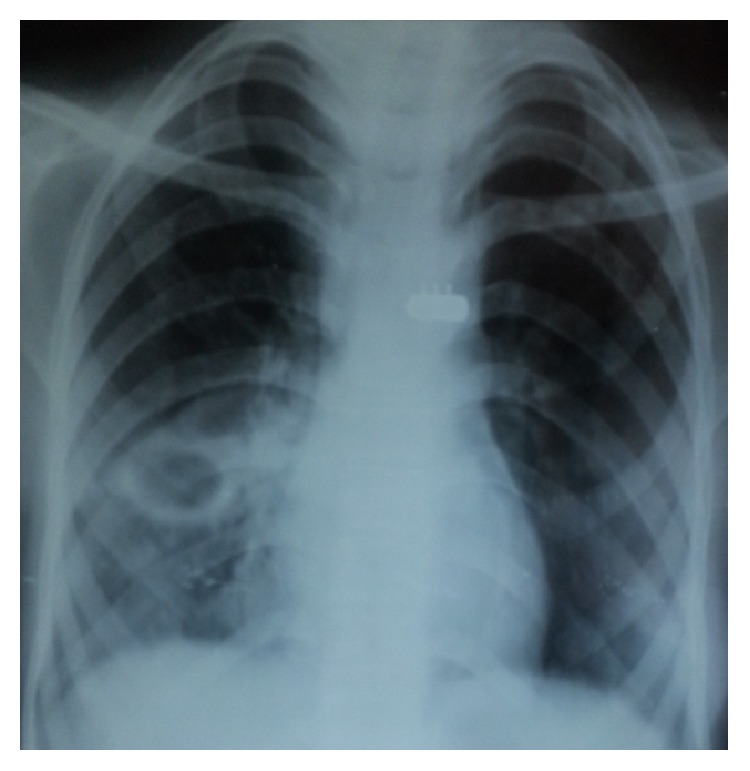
Chest X-ray showing far advanced lesion with cavity more than 4 cm in diameter.
